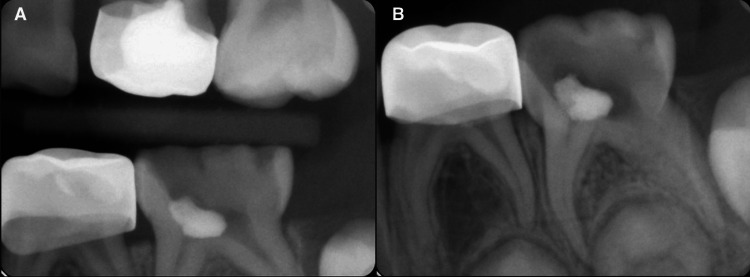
Preoperative periapical and bitewing radiographs showing broken #75 with remaining restoration from previous treatment A: Preoperative bitewing radiograph for #75; B: Preoperative periapical radiograph for #75
